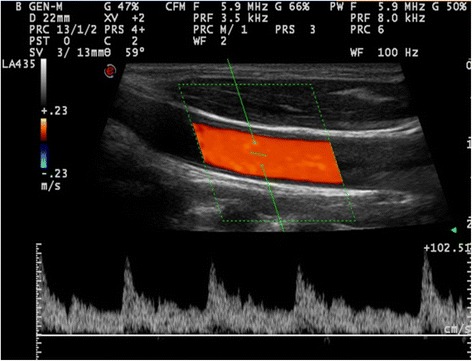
In spectral Doppler, the flow is displayed as a spectral waveform of changes in velocity during the cardiac cycle. The flow velocity is on the vertical axis with the time on the horizontal axis. The image is from a carotid artery
